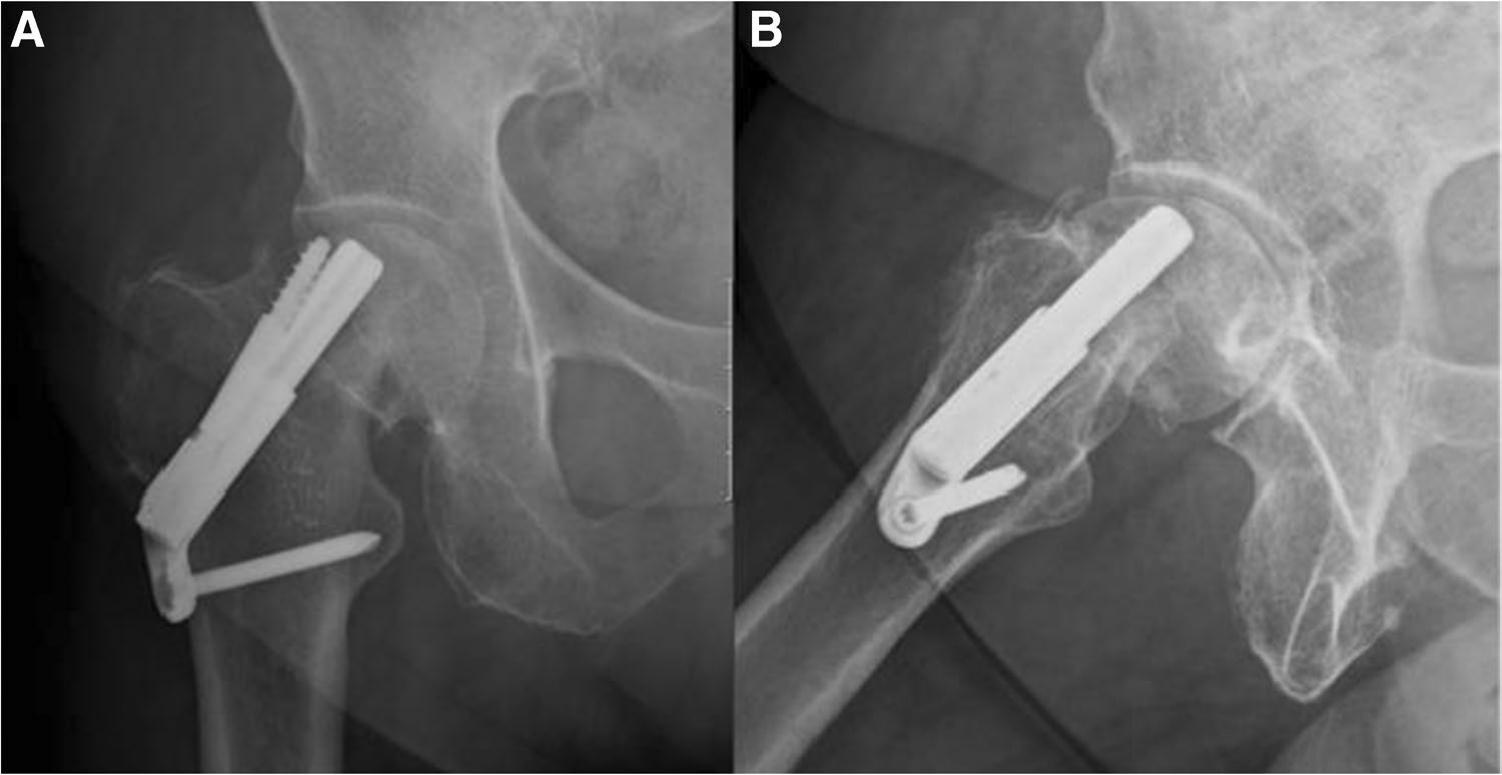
A, B AP and lateral radiographs 6 weeks after the surgical treatment. Radiographs demonstrate failure of fixation, cut-out of the implant. After the initial surgery, the patient was discharged home with instruction to full weight bear on the operated leg and was referred for physiotherapy treatment. Patient suffered from progressive hip pain and limp, walked short distance with the aid of a Zimmer frame. She was treated with revision surgery, hemiarthroplasty of the right hip
